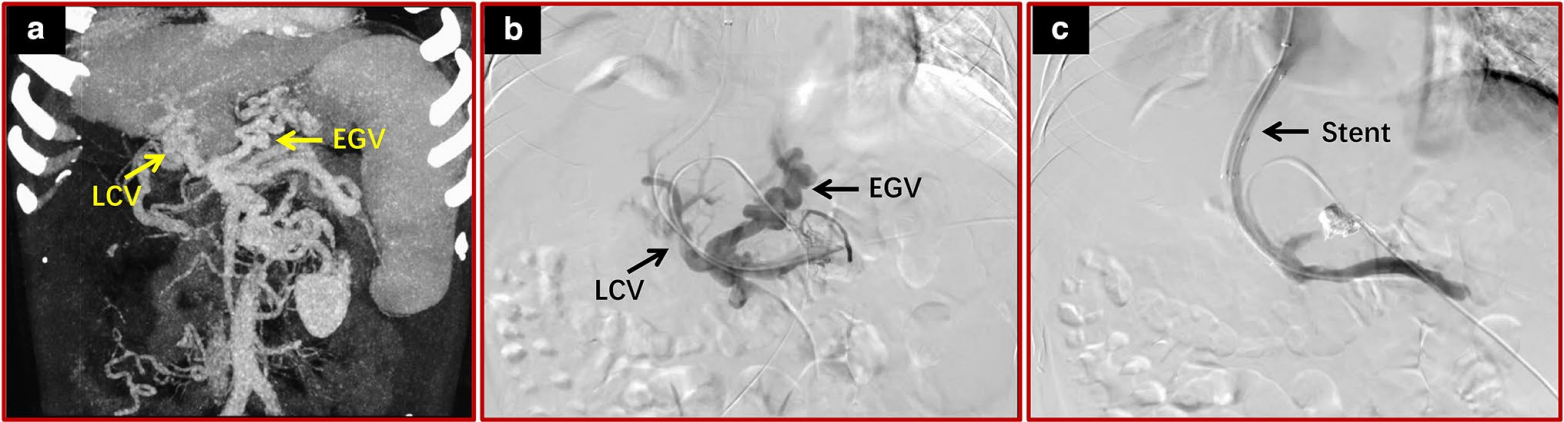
Using a collateral vessel that communicated with the variceal veins to establish a portosystemic shunt in a 50-year-old man with cirrhosis and cavernous transformation. a Spiral enhanced CT with multiplanar reconstruction showed cavernous transformation of the portal vein. The collateral vessels directly communicated with the variceal veins. b Direct portography confirmed that the targeted collateral vessels communicated with the variceal veins. c A stent was successfully placed between a large collateral vein and the right hepatic vein. Direct portography showed that the variceal veins had disappeared. *LCV* large collateral vessels, *EGV* esophageal and gastric varices
